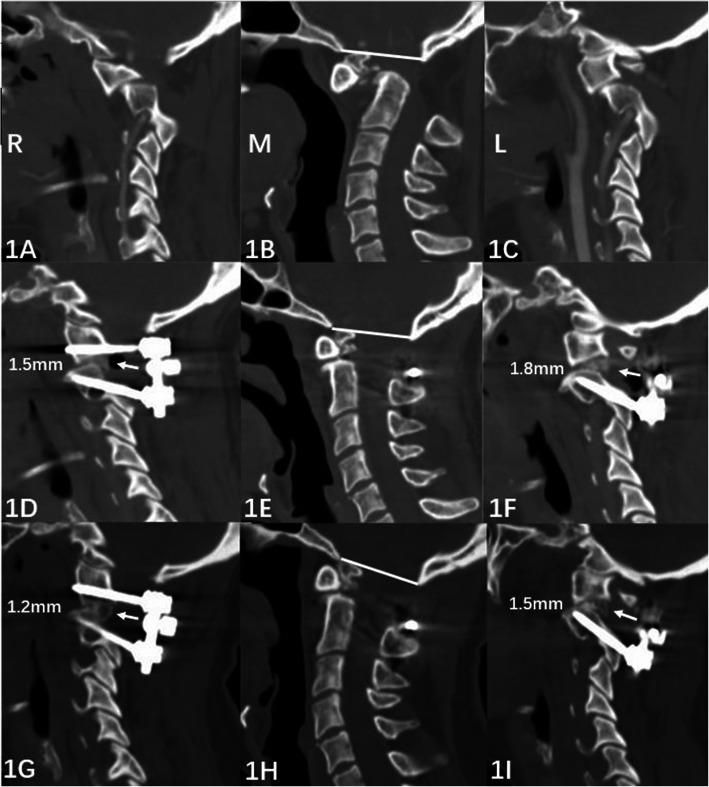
An atlantoaxial dislocation without BI managed with posterior nonstructural fusion. Female, 40 years. She sustained myelopathy (JOA score 11) due to atlantoaxial dislocation for 6 months and underwent atlantoaxial facet release, C1-2 fixation, reduction, and intraarticular nonstructural fusion via a posterior approach. At the final follow-up, her JOA score was 16. A–C Preoperative CT slice: right atlantoaxial facet joint (1A), midsagittal (1B, white line indicates Mcrea’s line), and left atlantoaxial facet joint (1C) views showed the relationship of C1 to C2, and there was no BI. D–F Immediate postoperative sagittal CT slice: nonstructural grafts (white arrow) within the atlantoaxial facet joints. The height of the joint space was 1.5 mm on the right side (1D) and 1.8 mm on the left side (1F). Midsagittal CT (1E) showed anatomical reduction of the dislocation. G–I CT slice at 6 months following surgery: grafts bridged the neighboring facet joints (white arrow) and suggested successful fusion. The height of the joint space was maintained well (1.2 mm on the right side, 1G; 1.5 mm on the left side, 1I). The reduction was maintained well (IH)
